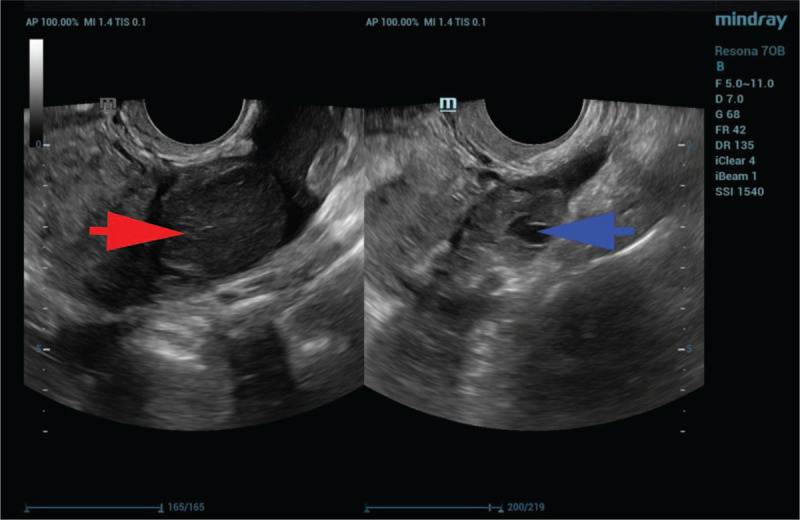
Transvaginal ultrasound before surgery confirmed masses in the bilateral adnexa regions. The red arrow indicates an EP in the right adnexa region. The blue arrow indicates the left adnexa region.
